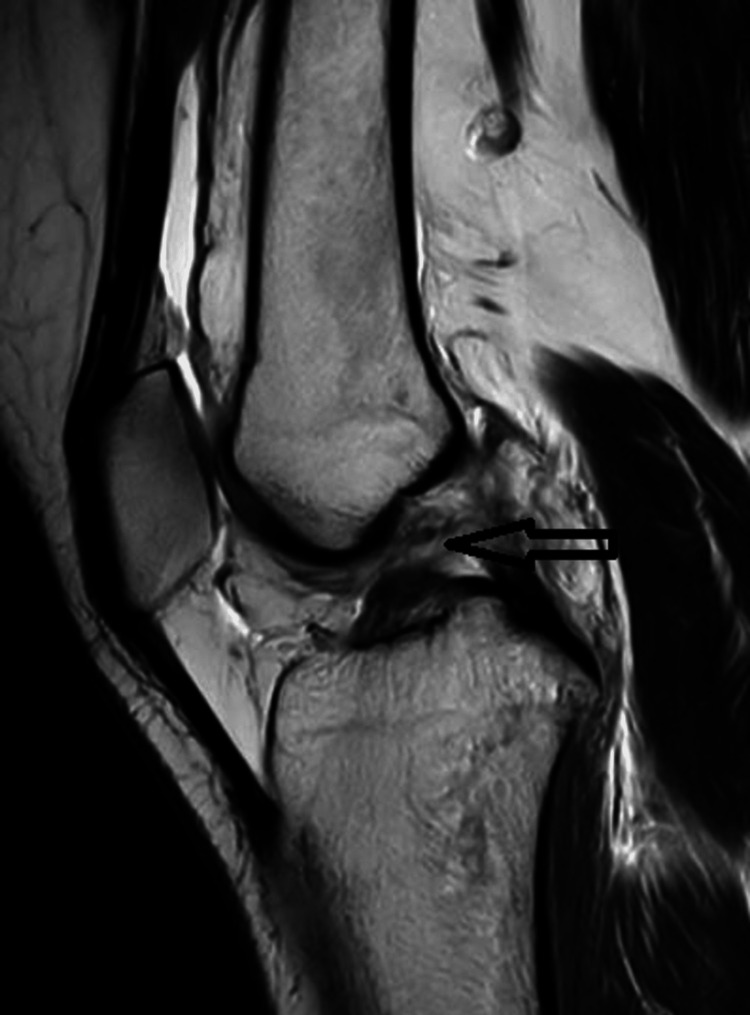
Left knee MRI image The image shows a complete ACL tear with a medial meniscus tear.
